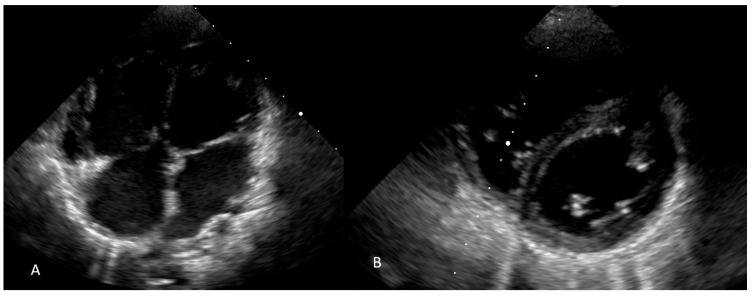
Postoperative echocardiography: (A) 4 chamber view; and (B) short axis view at the ventricles.
